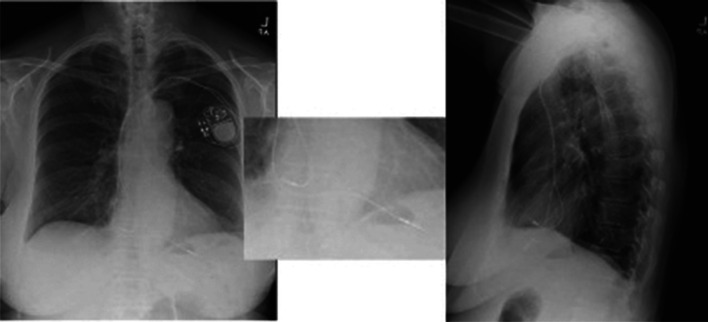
Chest X-ray at initial presentation. Anteroposterior and lateral view demonstrating evidence of pacemaker leads in the right atrium and right ventricle. A close-up of the pacemaker leads is shown in the center image.
